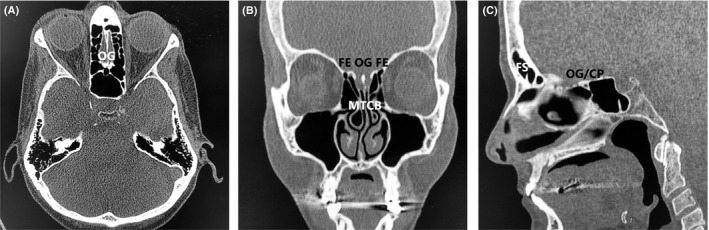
A, Axial CT view of normosmic patient showing well‐developed olfactory grooves (OG). B, Coronal CT view of normosmic patient showing normal fovea ethmoidalis (FE), well‐developed olfactory grooves (OG), and incidental middle turbinate concha bullosa (MTCB). C, Sagittal CT view of normosmic patient showing a well‐developed cribriform plate (CP) with well‐developed olfactory grooves (OG) and clearly visible fenestrations for olfactory filaments with well‐pneumatized frontal sinuses (FS)
